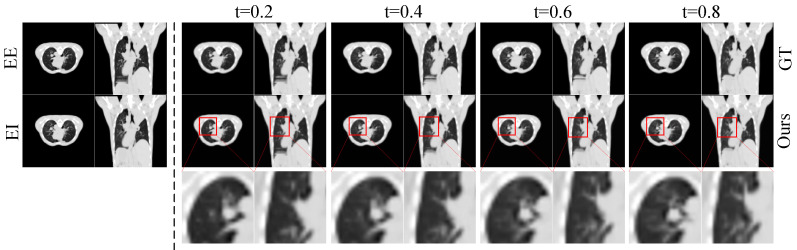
Visualization of lung CT interpolation results at different time points.
